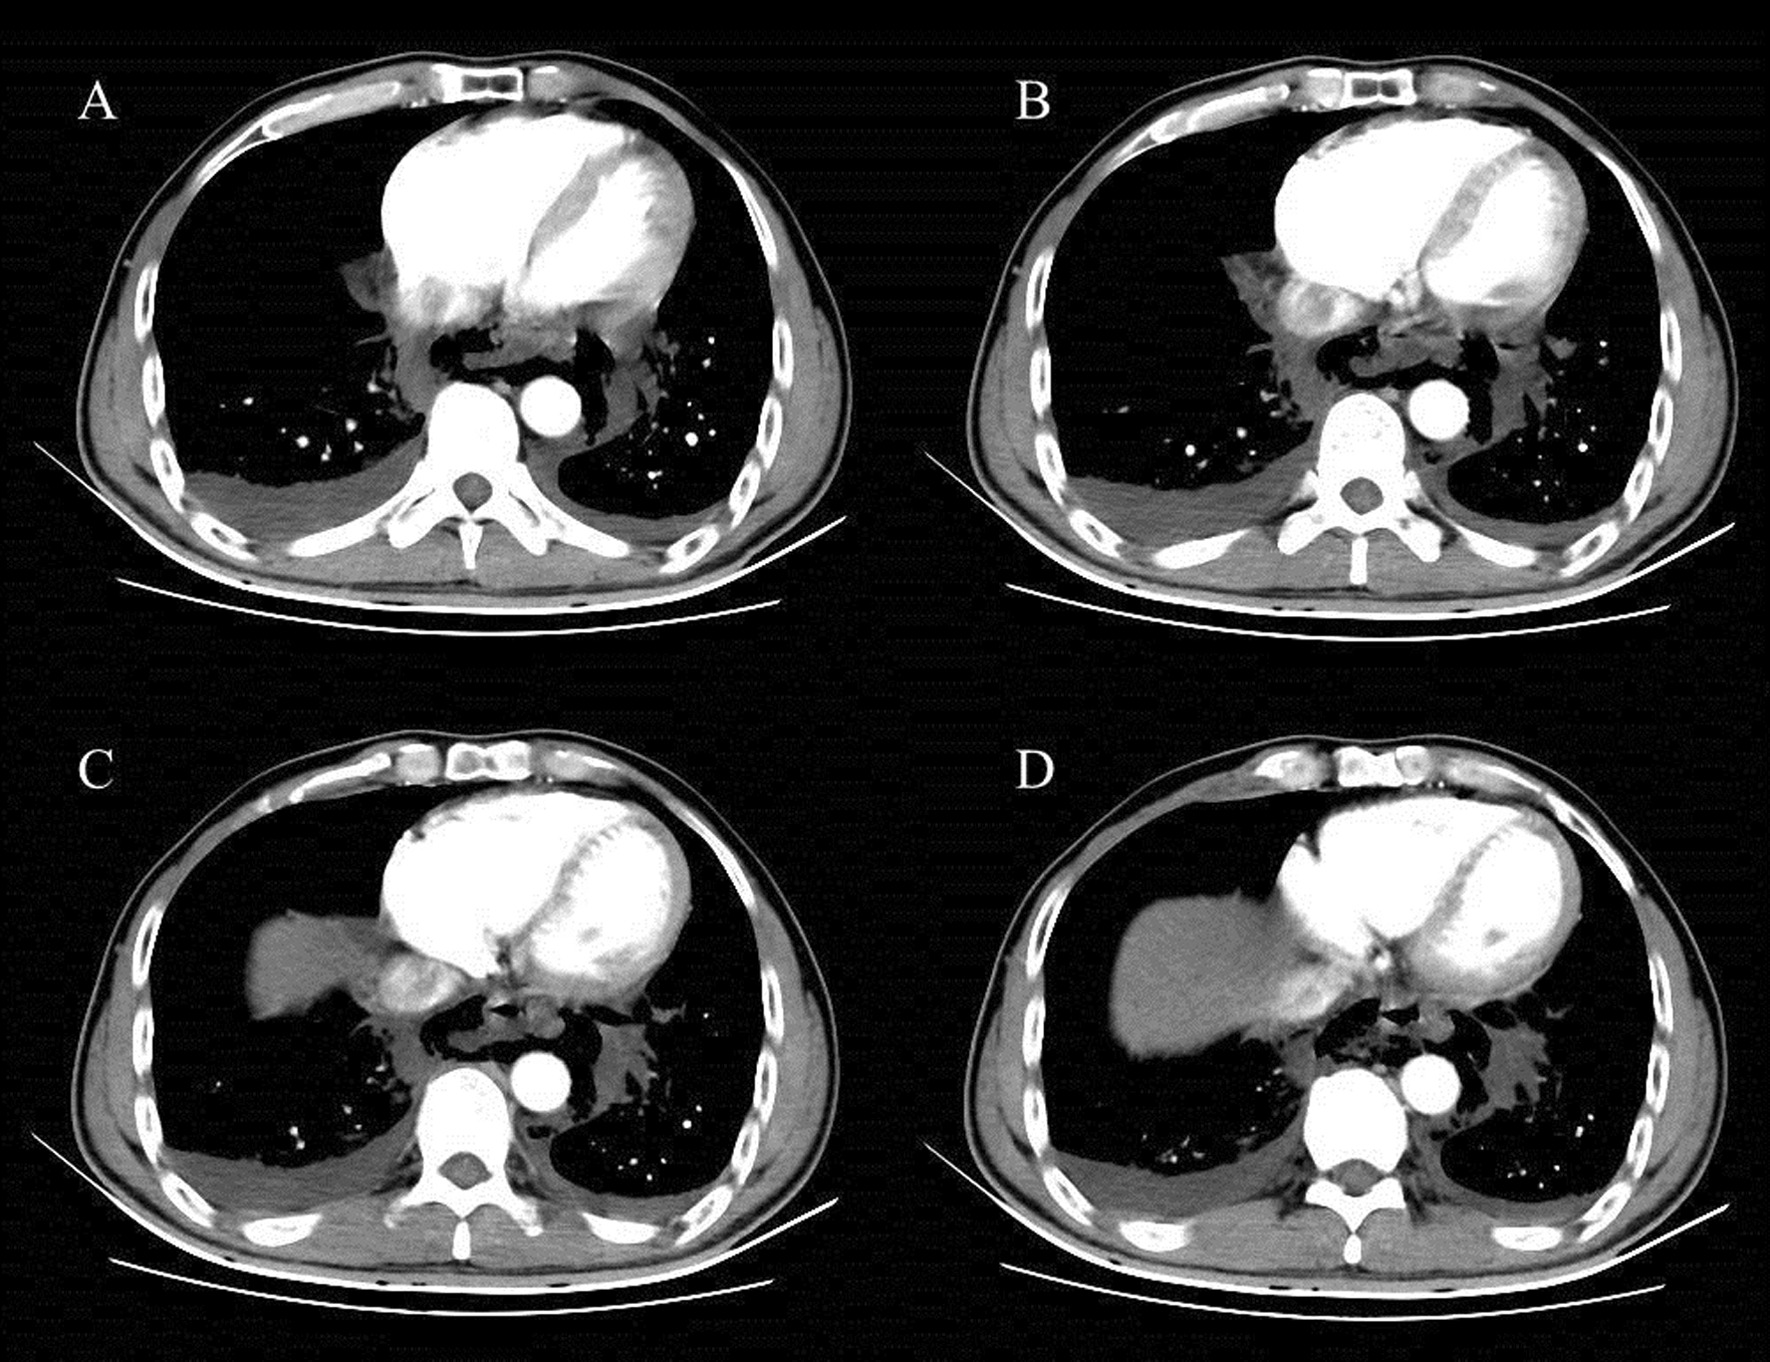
CT showing esophageal rupture with air leakage into the mediastinum and heterogeneous pleural effusion
